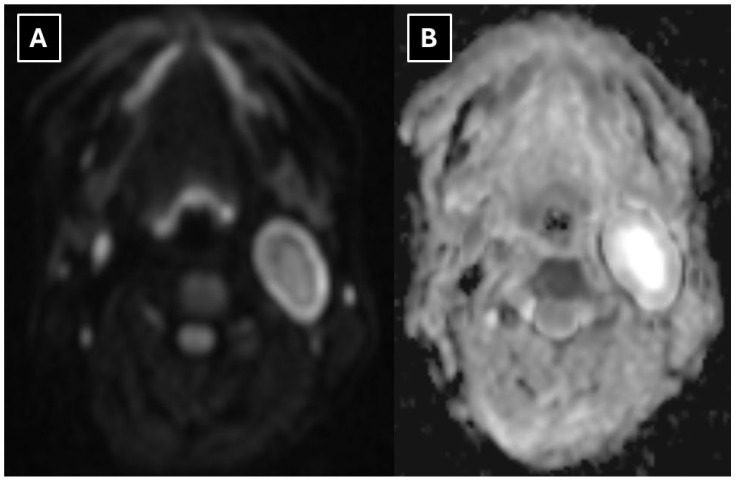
MRI scan of the neck. (A) Axial TRACE map of DWI b1000 and (B) corresponding apparent diffusion coefficient (ADC) map show mild diffusion restriction in the solid peripheral components and facilitated diffusion internally, secondary to cystic degeneration. The diffusion pattern is suggestive of a benign nerve sheath tumor.
